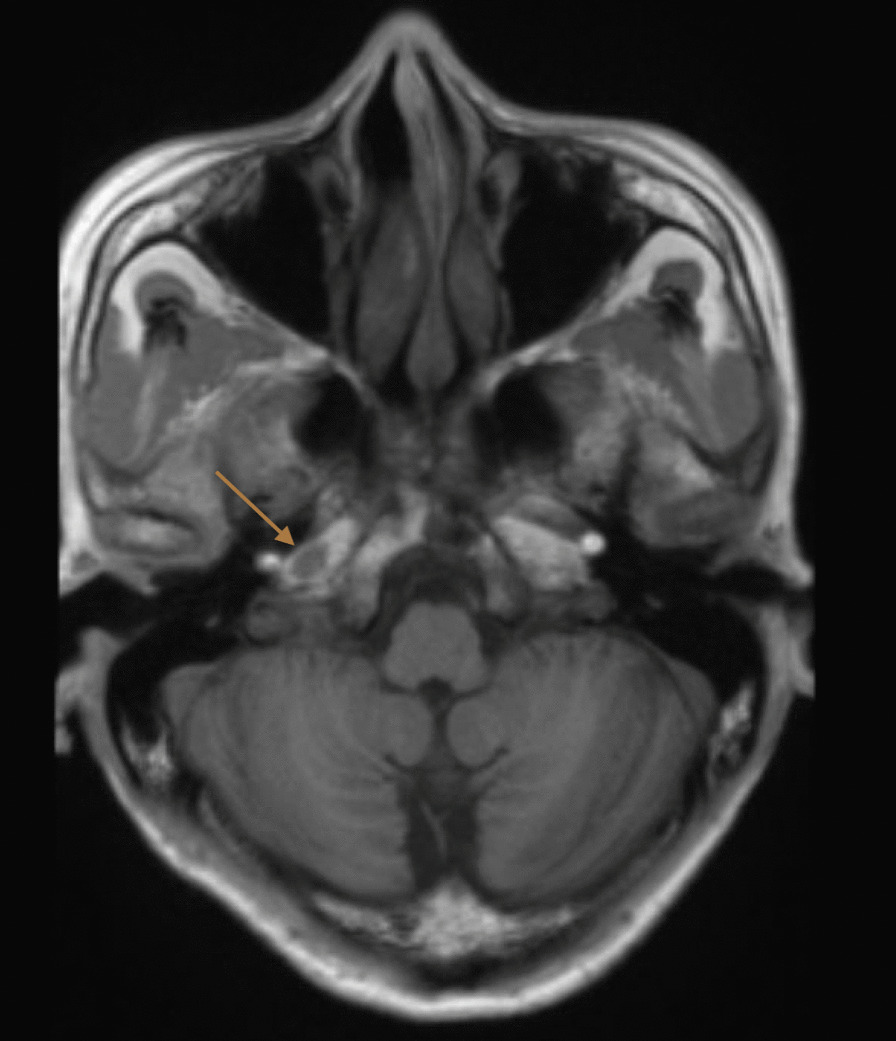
Magnetic resonance imaging showing an oval-shaped, nonspecific isointense T1 signal (arrow) near the right petrous apex
